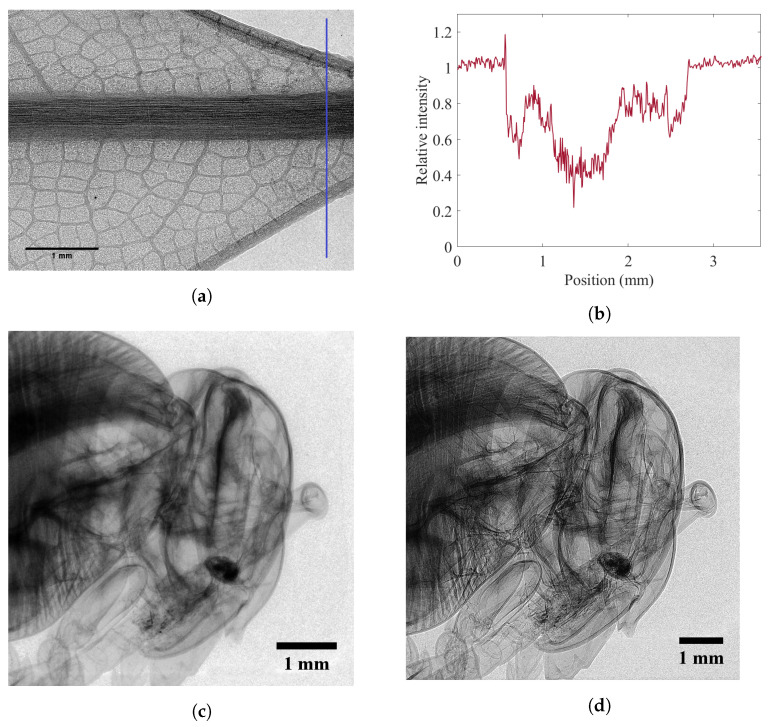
(a) Phase-contrast image of a dried bay leaf using a prototype version of the BrillianSe™ X-ray detector and a microfocus source at 60 kV${}_{p}$, $z_{so}$ = 18 cm, and $z_{od}$ = 8 cm; (b) profile of relative intensity along the vertical cross-section (blue line); (c) X-ray attenuation image of a bee head imaged at 60 kV${}_{p}$, $z_{so}$ = 18 cm, and $z_{od}$ = 1 cm; (d) X-ray phase-contrast image of the same bee head imaged at 60 kV${}_{p}$, $z_{so}$ = 18 cm, and $z_{od}$ = 8 cm.
